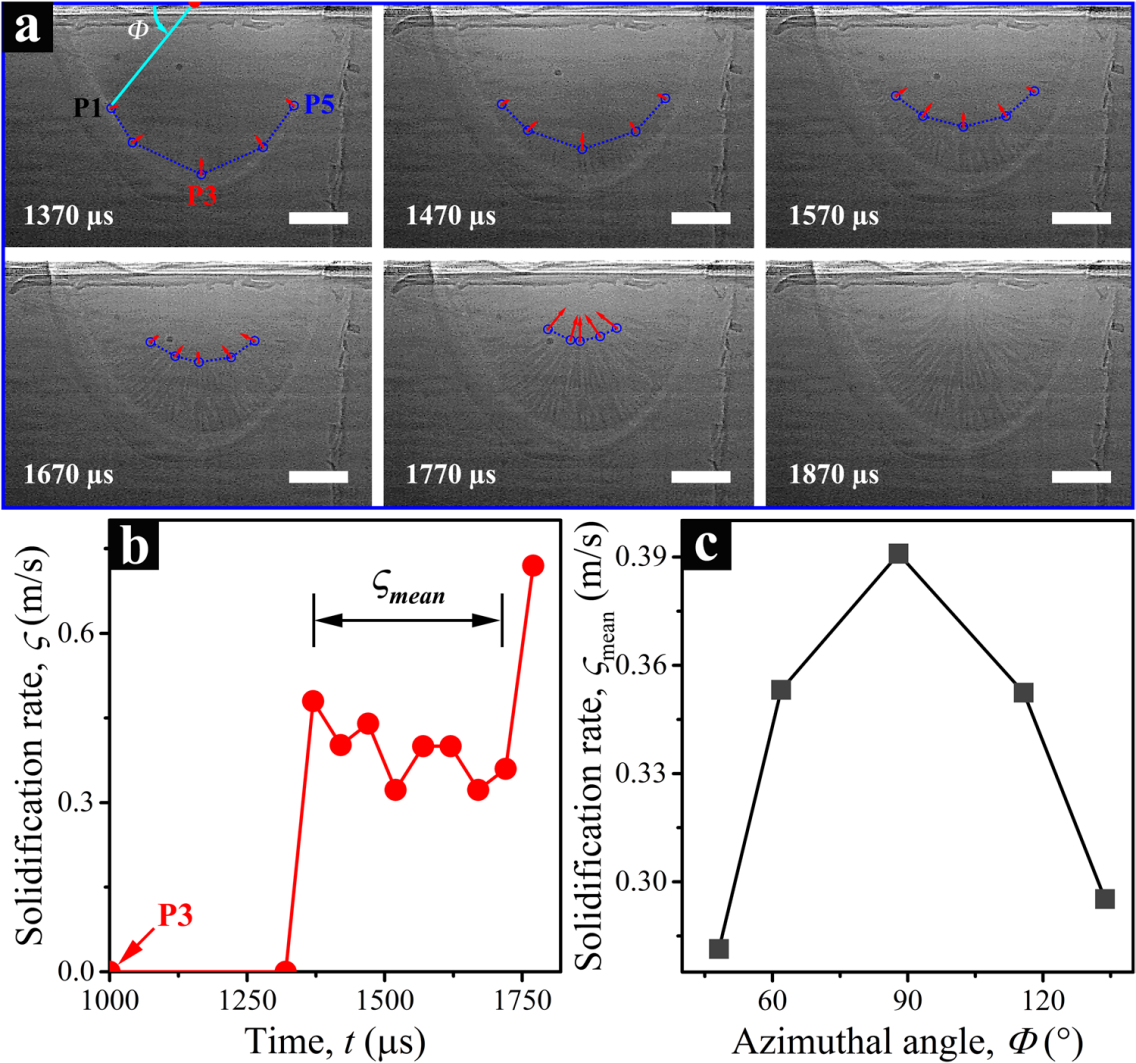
Measurement of the solidification rate of a Ti-6Al-4V plate sample in laser melting process. (a) Dynamic X-ray images of the solidification process collected at *t* = 1370, 1470, 1570, 1670, 1770, and 1870 µs. Note the laser was turned off at *t* = 1000 µs. The red dot at the top of (a) locates the center of the melt pool. The blue circles are the target points on the liquid-solid interface for tracking, and are connected by blue dotted lines to outline the melt pool boundary. The red arrow on each spot indicates the solidification velocity. All the scale bars are 100 µm. (b) Solidification rate of target point P3 as a function of time. The solidification rate is calculated over the time unit of 50 µs. (c) Mean solidification rate as a function of the azimuthal angle. The mean solidification rate is averaged over the equilibrium growth period shown in (b), and the azimuthal angle is defined in (a).
